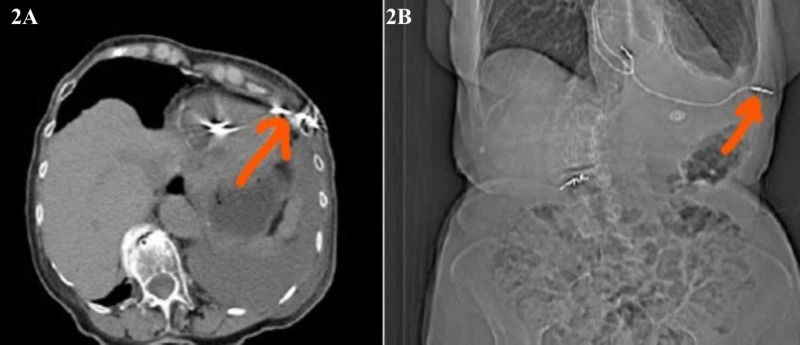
CT scan showing abnormal coronal and sagittal section position of ventricular misplaced lead. (A) CT scan showing coronal section of the misplaced ventricular lead. (B) CT scan showing sagittal section of the misplaced ventricular lead.
